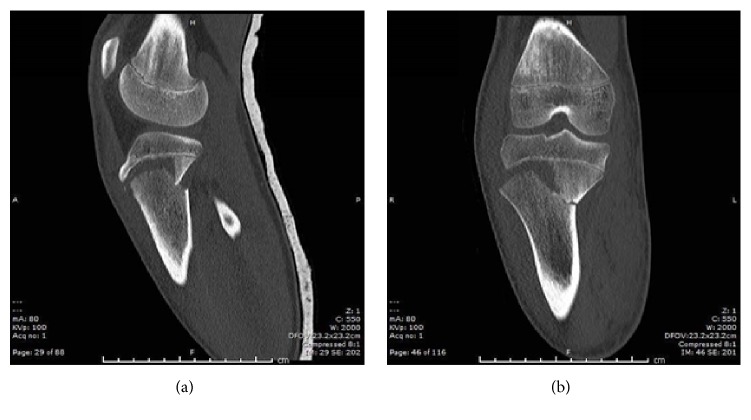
Sagittal (a) and coronal (b) slices of noncontrast CT scan of patient's right knee demonstrating grade IV proximal tibial avulsion fracture.
